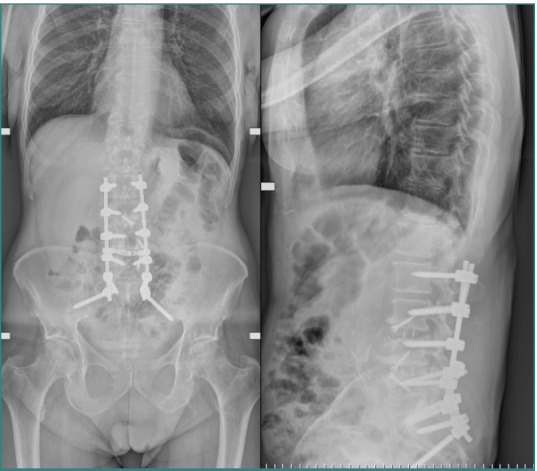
Postoperative F&P X-rays depicting posterior spinal fusion from L2 to S1 with ALIF at L4-L5 and L3-L4 levels
